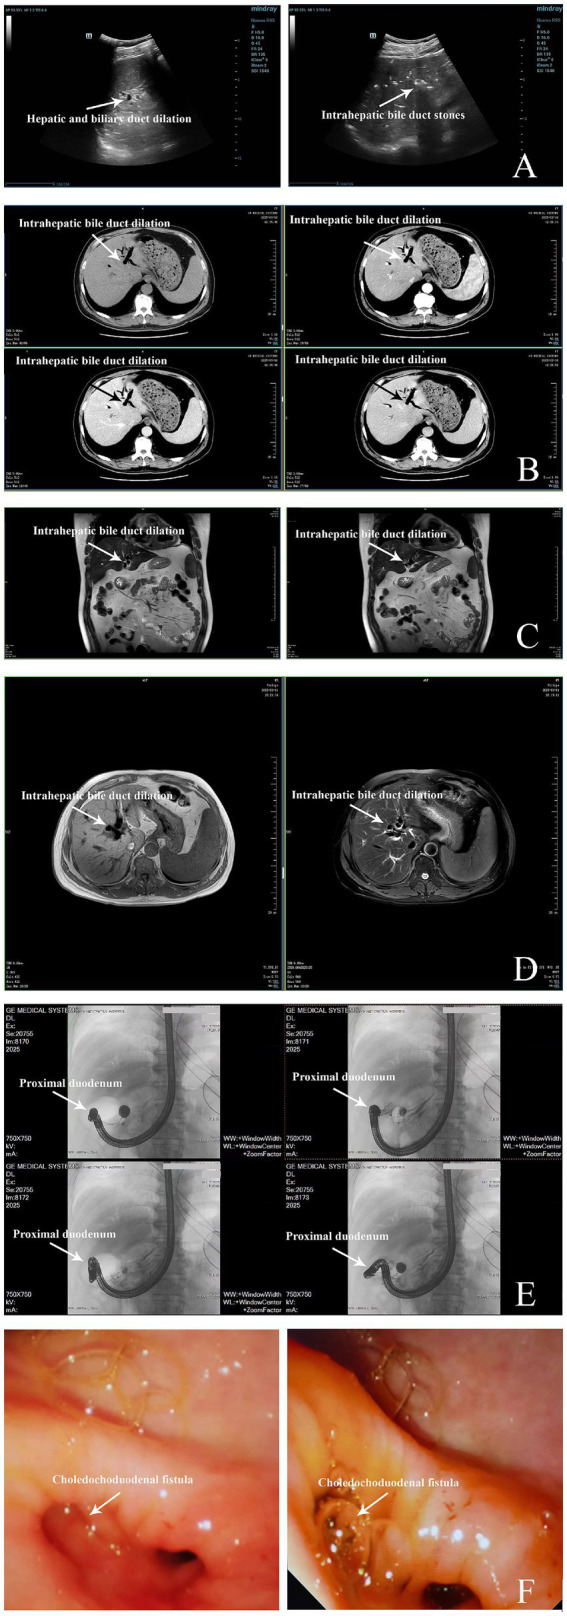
Imaging findings of gallbladder and related conditions. (A) Color Doppler ultrasound of hepatobiliary system (7 days before diagnosis): Ultrasound demonstrates heterogeneous hepatic echogenicity with anterior hyperechoic density and posterior attenuation, consistent with mild steatosis. Multiple beaded hyperechoic foci (largest 1.5 × 0.5 cm) with posterior shadowing suggest intrahepatic biliary calculi or pneumobilia. Post-cholecystectomy anatomy with a non-dilated proximal common bile duct (0.5 cm) and splenomegaly (length 12.1 cm, thickness 4.3 cm). Color Doppler flow imaging (CDFI) reveals preserved hepatic and splenic vascular flow, consistent with non-obstructive pathology. (B) Contrast-enhanced CT of Hepatobiliary, Pancreatic, and Splenic Systems (7 days before diagnosis): Axial CT demonstrates intra- and extrahepatic biliary pneumobilia and dilation, a non-visualized gallbladder (post-cholecystectomy), hepatic hyperdense nodules, and mild splenomegaly. The pancreas appears unremarkable. Measurements: hepatic nodule size up to 0.5 cm; splenic length ~12 cm. (C) Non-contrast MRCP (6 days before diagnosis): Magnetic resonance cholangiopancreatography reveals mild intrahepatic bile duct dilatation with pneumobilia, absent gallbladder (post-cholecystectomy), and splenomegaly. Pancreatic ductal anatomy preserved, no choledochal or pancreatic duct obstruction. (D) MRCP (5 days before diagnosis): MRCP confirms intrahepatic bile duct dilation (IHBRD) with pneumobilia, absent gallbladder, non-dilated choledochus and pancreatic duct, and no intraductal filling defects. (E) ERCP (on diagnosis date, March 17, 2025): Endoscopic retrograde cholangiopancreatography demonstrates a choledochoduodenal fistula with two inflammatory strictures at the distal duodenum and fistula orifice. Balloon dilation unsuccessful, bile drainage limited. (F) Intraoperative Endoscopy (3 days after diagnosis, March 20, 2025): Endoscopic view during laparoscopic surgery reveals choledochoduodenal fistula between the common bile duct and duodenal bulb with an inflammatory stricture and active bile leakage at the fistula site.
